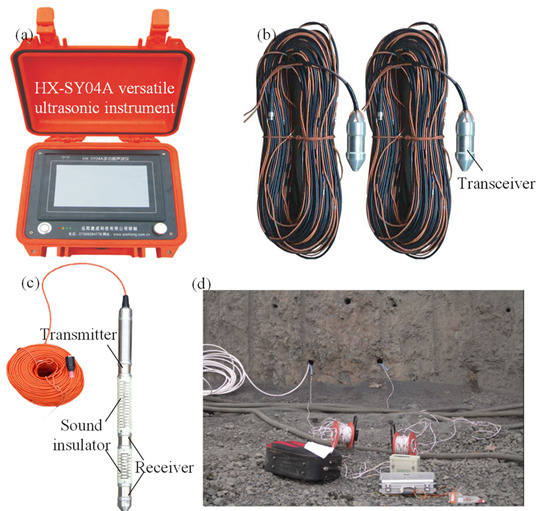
Sonic test system: (a) HX-SY04A versatile ultrasonic instrument; (b) Cross-hole transducer; (c) Single-hole transducer; (d) On-site sonic test.
